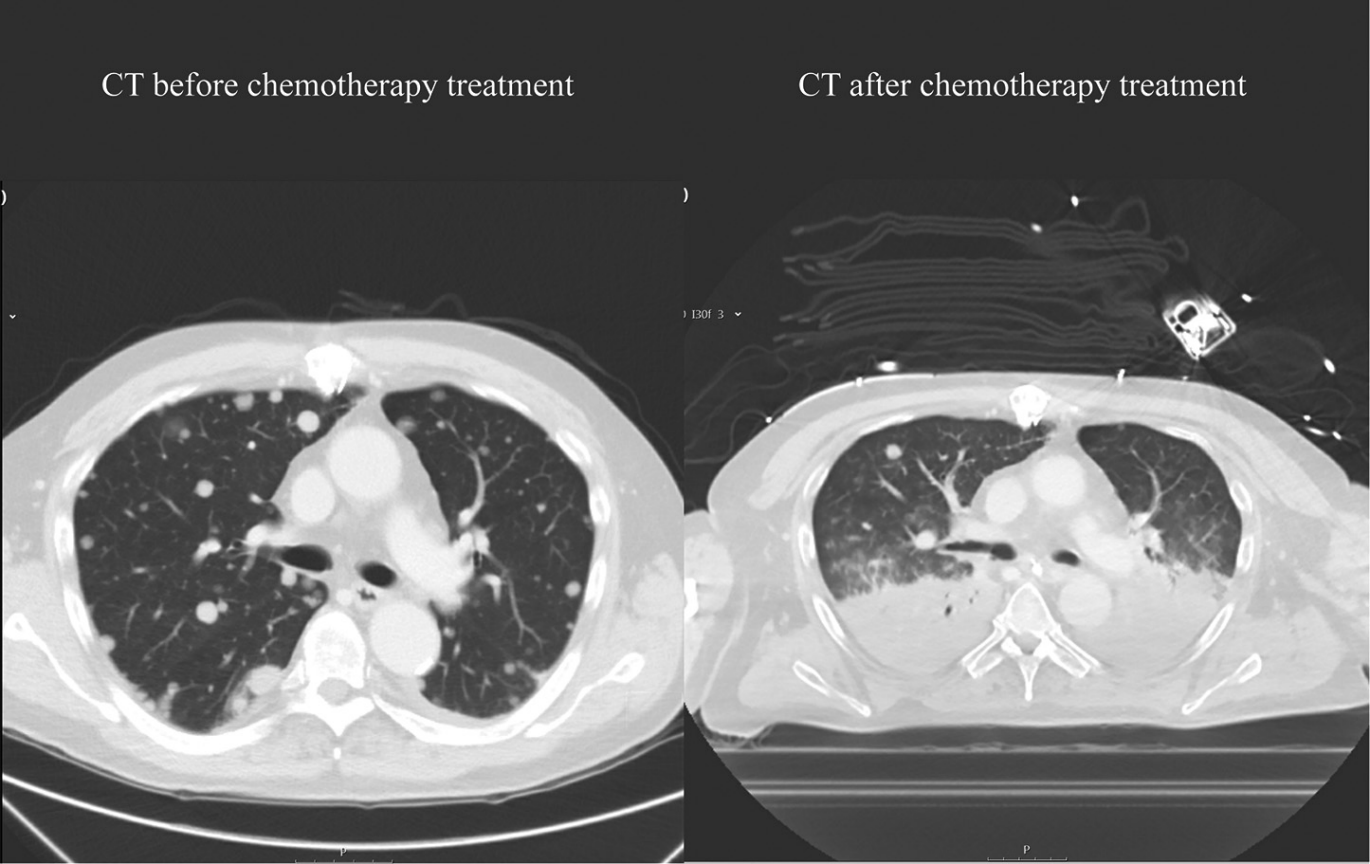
Chest CT Scan Before and After Initiation of Chemotherapy Chest computed tomography (CT) scan before chemotherapy showing extensive pulmonary metastases with enlarged mediastinal lymph nodes (left). On the day 11 after chemotherapy initiation, a chest CT post-cardiac arrest showed bilateral infiltrates in the lungs and regression of pulmonary metastases (right).
